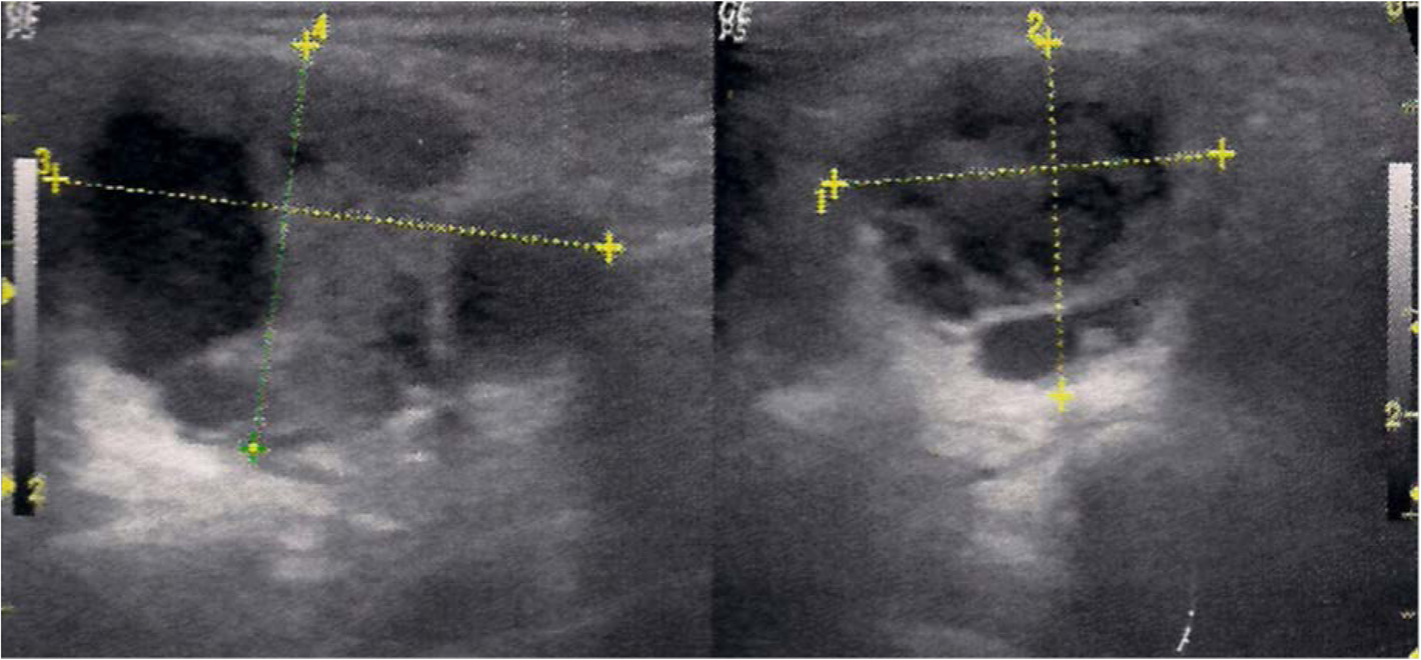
Ultrasonography revealed a mixed component nodule of the left parotid
